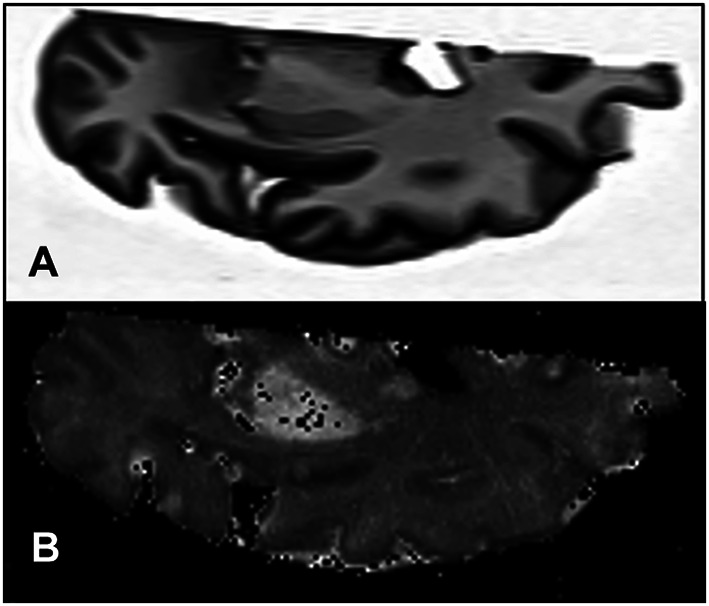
MRI scan showing an axial T1-weighted image of a one cerebral hemisphere, and b the corresponding *R*_2_* map. The bright areas in b are the putamen and the globus pallidus with higher *R*_2_* rates as a consequence of age-related iron accumulation
